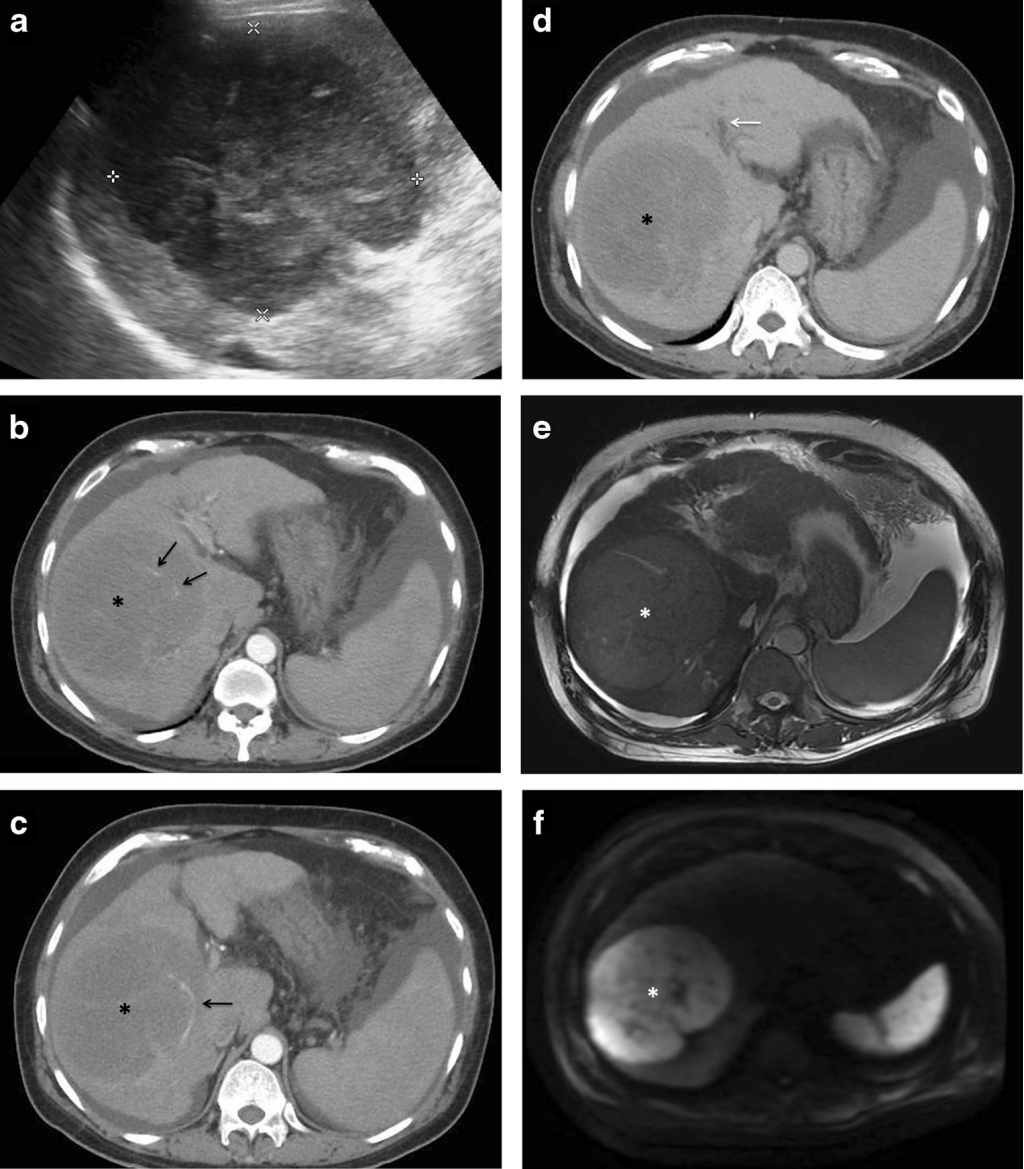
Primary hepatic non-Hodgkin lymphoma in a 55-year-old man with jaundice. a Gray-scale US image showing a large, well-defined, markedly hypoechoic lesion in the right lobe of liver (marked by calipers). CECT images (b-d) demonstrating a hypoenhancing lesion (asterisks) with arterial channels coursing through it (arrows in b and c). Changes of liver cirrhosis and ascites can also be seen with biliary dilatation (arrow in d). MR images reveal a homogeneous, mildly T2-hyperintense, diffusion restricting lesion (asterisk in e and f)Occasionally, a central “scar” can be seen in this type of hepatic lymphoma, mimicking focal nodular hyperplasia [33] (Fig. 5). However, poor enhancement of the lesion on all the contrast-enhanced phases allows one to confidently differentiate between the two entities.
